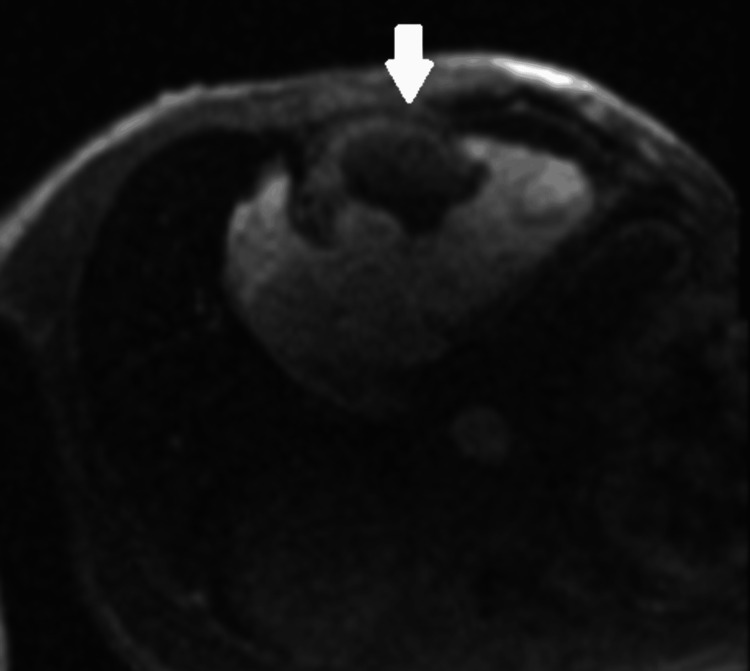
Perfusion scan in cardiac MRI showed subtle heterogeneous enhancements, highly favouring the diagnosis of metastases rather than thrombus.
